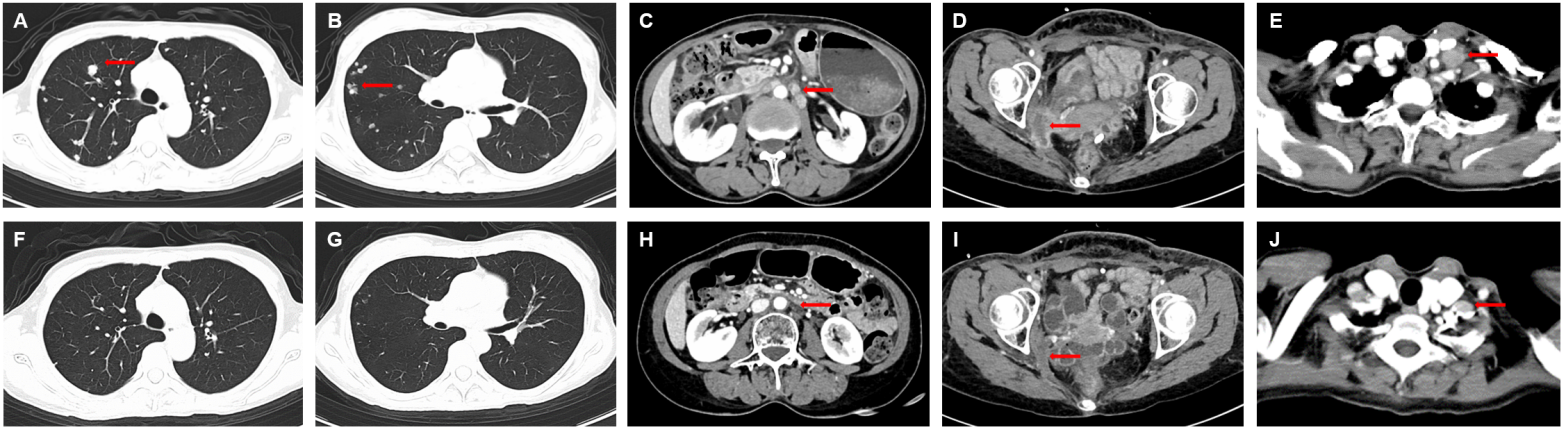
Computed tomography scan before and after Disitamab vedotin combined with toripalimab treatment. (A-E) Lung, pelvic lymph node and left supraclavicular lymph nodemetastases before treatment with Disitamab vedotin in combination with toripalimab. (F-J) All lesions were significantly reduced after 18 cycles of combined treatment. The red arrows indicate the lesions.
